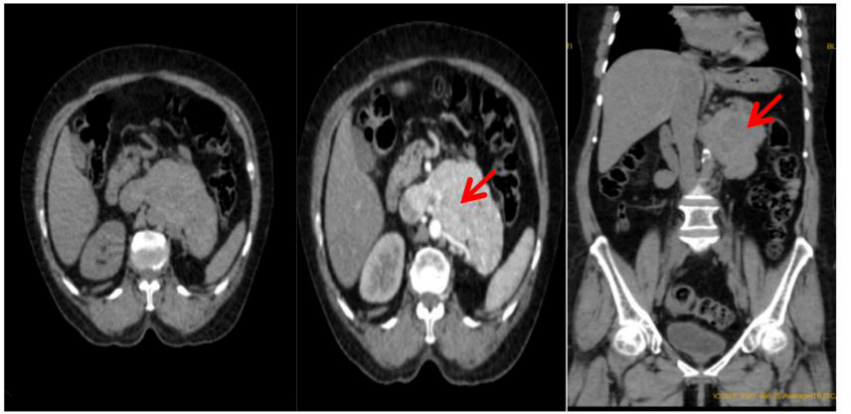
Contrast CT showing heterogeneously enhancing mass in the left retroperitoneum with intrinsic foci of necrotic areas with involvement of periaortic lesion and extension into the inferior vena cava.
